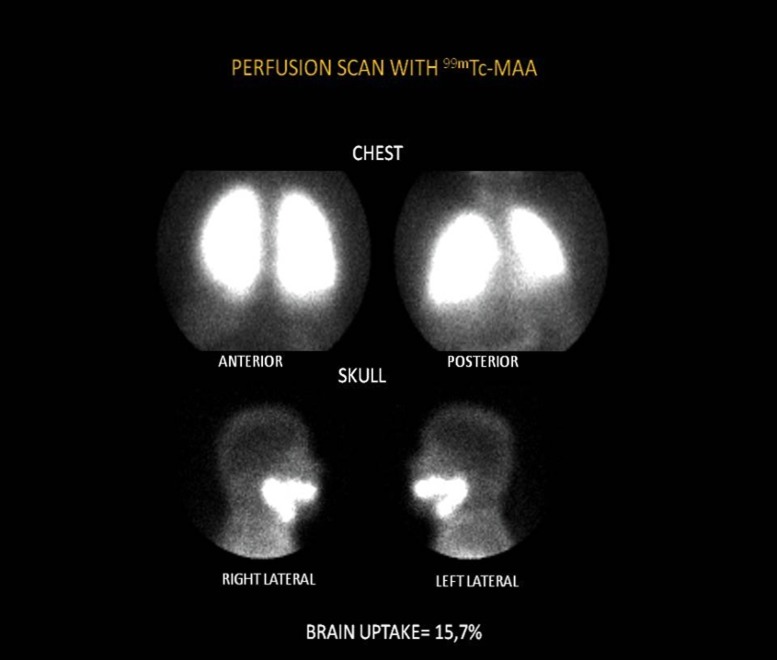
Anormal scintigraphy. Brain uptake = 15.7%.
